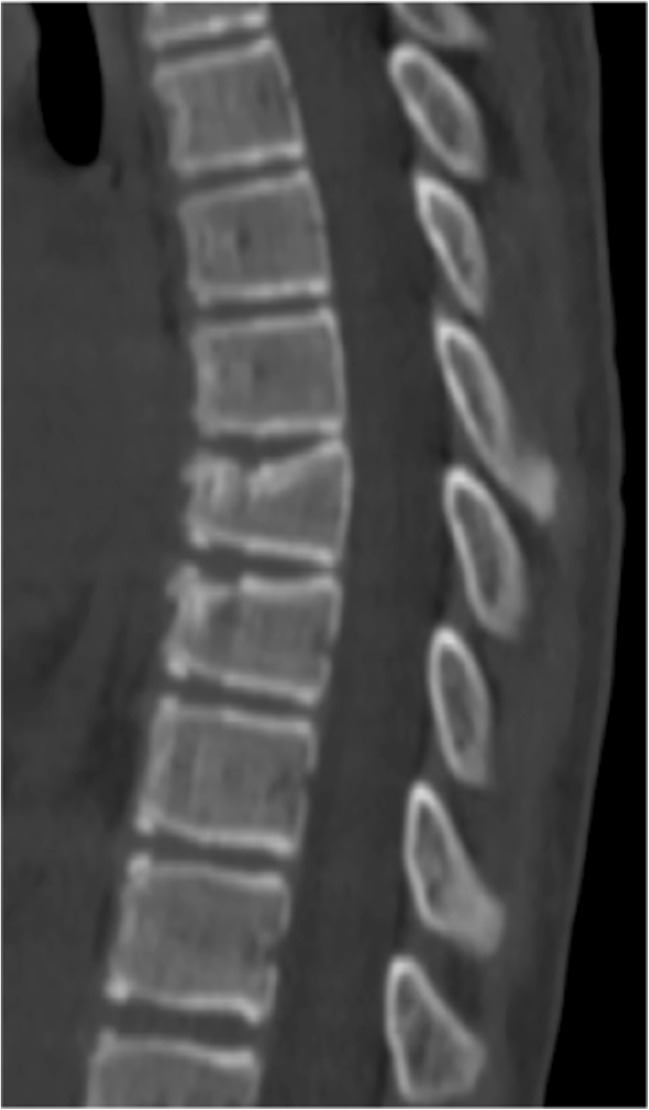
CT scan of fractured VB (sagittal)
